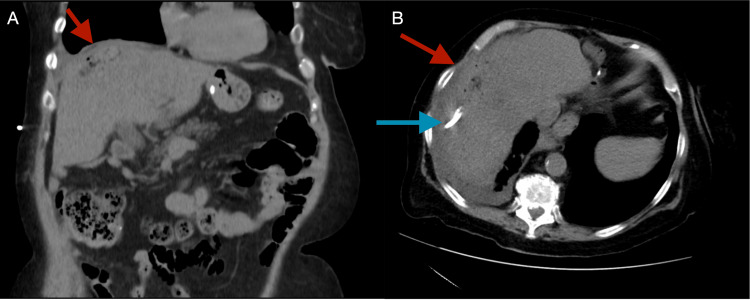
CT of the abdomen without contrast enhancement performed three weeks after drain insertion showing near resolution of the hepatic fluid collection (red arrows) with the drain (blue arrow) before it was removed the same day. (A) Coronal view. (B) Axial view. CT: computed tomography
